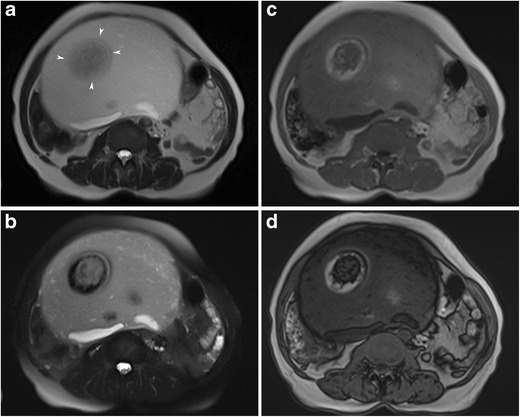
Axial T2-weighted (a), fat-saturated T2-weighted (b), in-phase (c) and opposed-phase T1-weighted (d) MR images of a 56-year-old female with a floating ball (arrowheads) in a mature cystic teratoma. The central core of the ball contains minor fat, which is seen as decreased signal intensity in the opposed-phase image, and an outer layer with a major fat signal that decreases in the fat-saturated T2-weighted image
